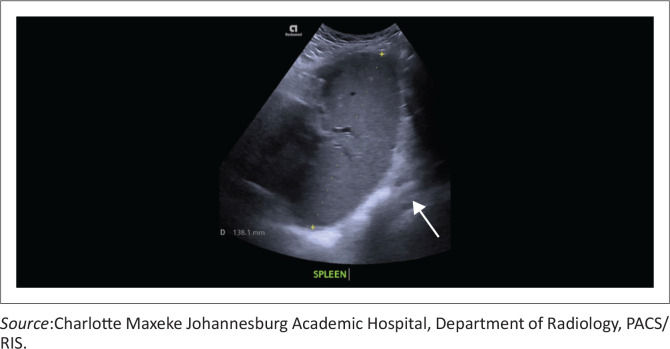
Greyscale ultrasound demonstrating splenomegaly, with the spleen in the left upper quadrant measuring 13.8 cm in craniocaudal dimension.
